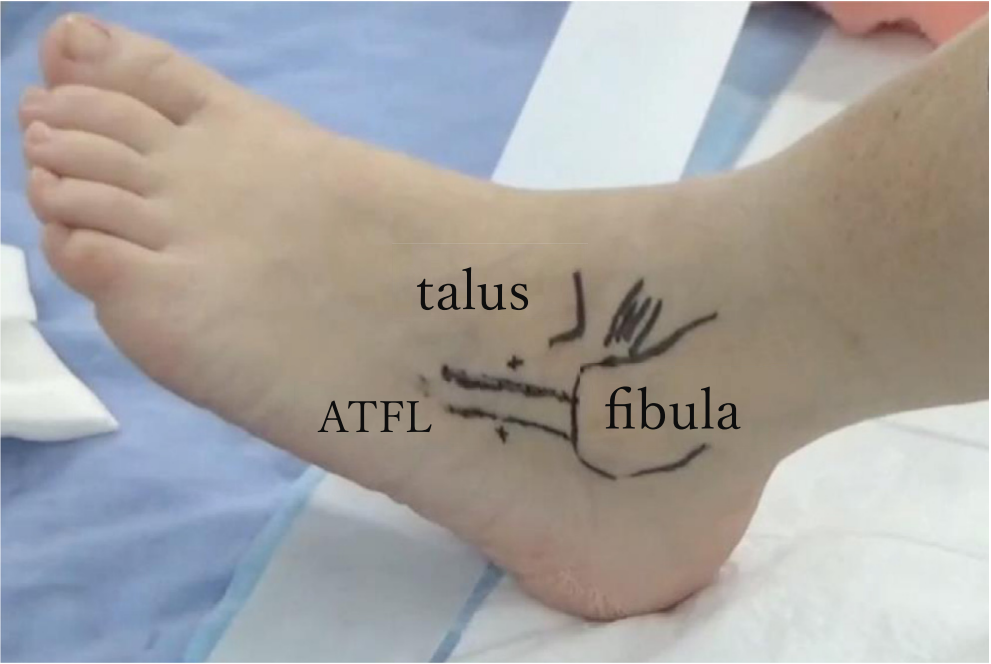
Patient's left leg landmarks showing anterior talofibular ligament (ATFL), fibula, and talus created using ultrasonography. The course of the ATFL is continuous with the fibula but is separated on the talar side and runs laterally. The far accessory anterolateral portal is marked distal to the fibula and on the talar side of the ATFL.
